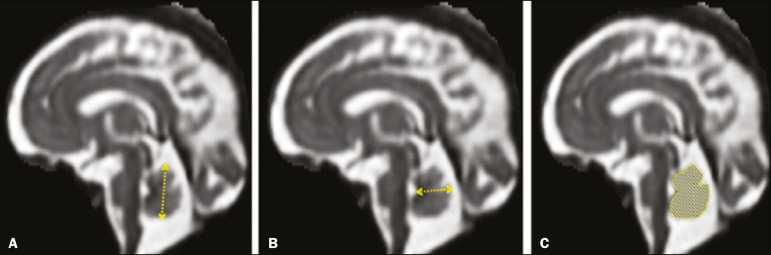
MRI measurements of cerebellar vermis height (A), width (B), and area (C).
